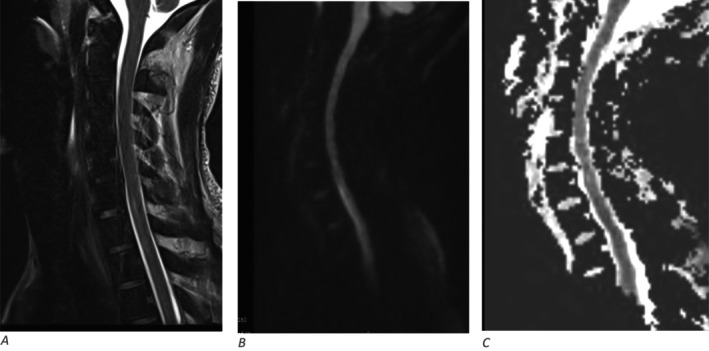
Linear area of T2 hyper‐intensity (A) with suspected diffusion restriction in DWI (B) and hypo‐intensity in ADC image (C) is seen at the anterior spinal cord at C6–C7 level suggestive of infarct.
